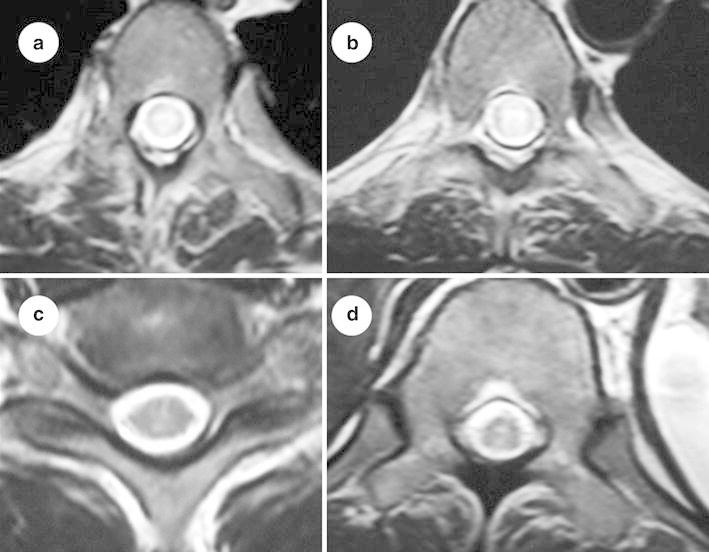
T2-weighted MRI of thoracic spine (axial section) showing poorly delineated intramedullary hyperintense lesion (a, b, c, d). There is no cord expansion
